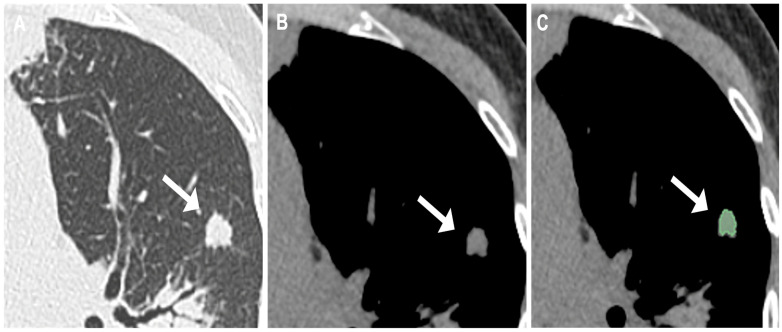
(A) Lung window and (B) mediastinal window images from a non-contrast chest CT of a 63-year-old female patient. Images show small solid nodule in left superior lobe (arrows) with pathologically proven diagnosis of granuloma. (C) Radiomics volumetric segmentation (highlighted in green).
